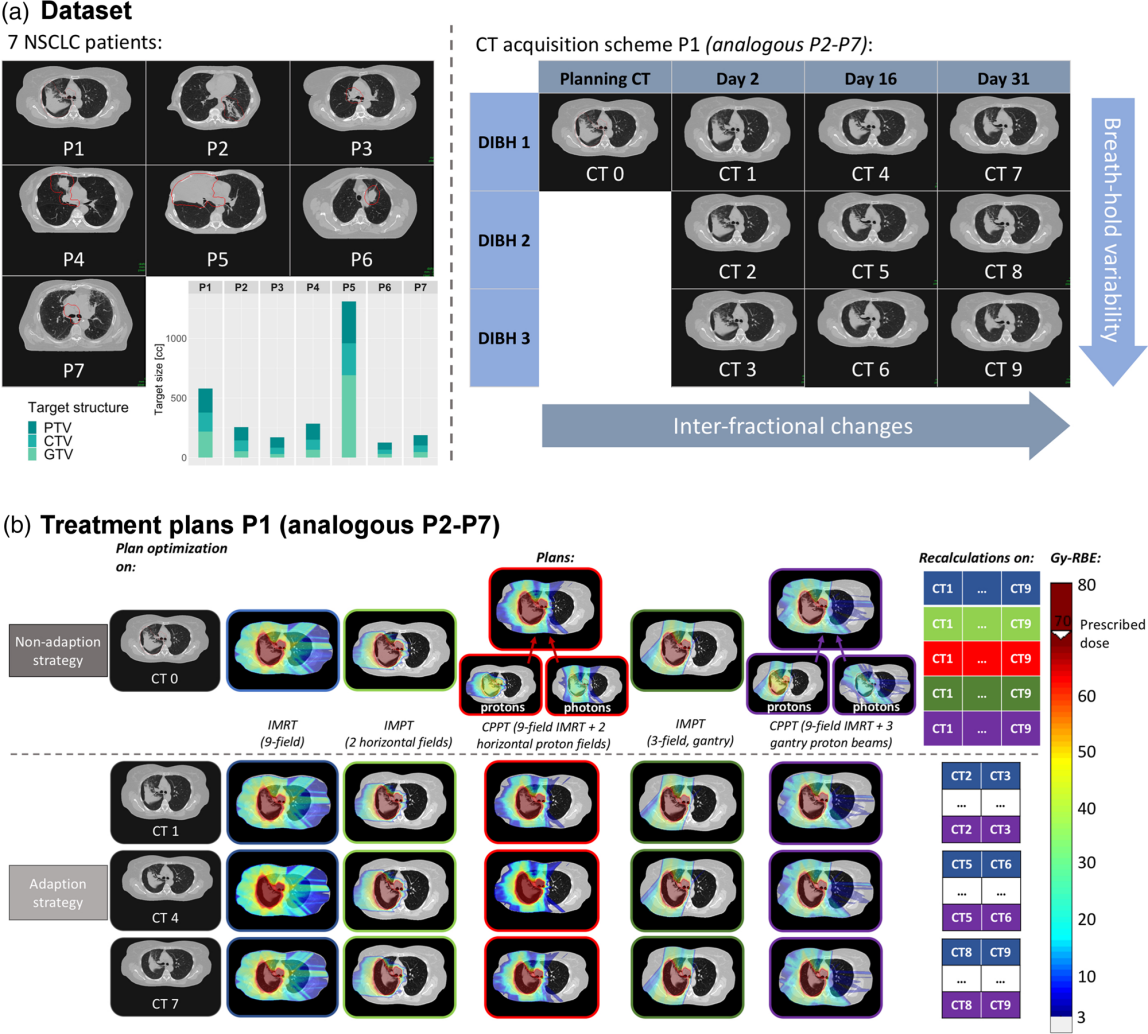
(a) Overview of the dataset including an example slice for each of the seven patients together with the target structure sizes for the different patients. The CT acquisition scheme is shown on the example of patient 1. (b) Optimized treatment plans for patient 1 together with the recalculation scheme for the non‐adaptive and the adaptive regime
